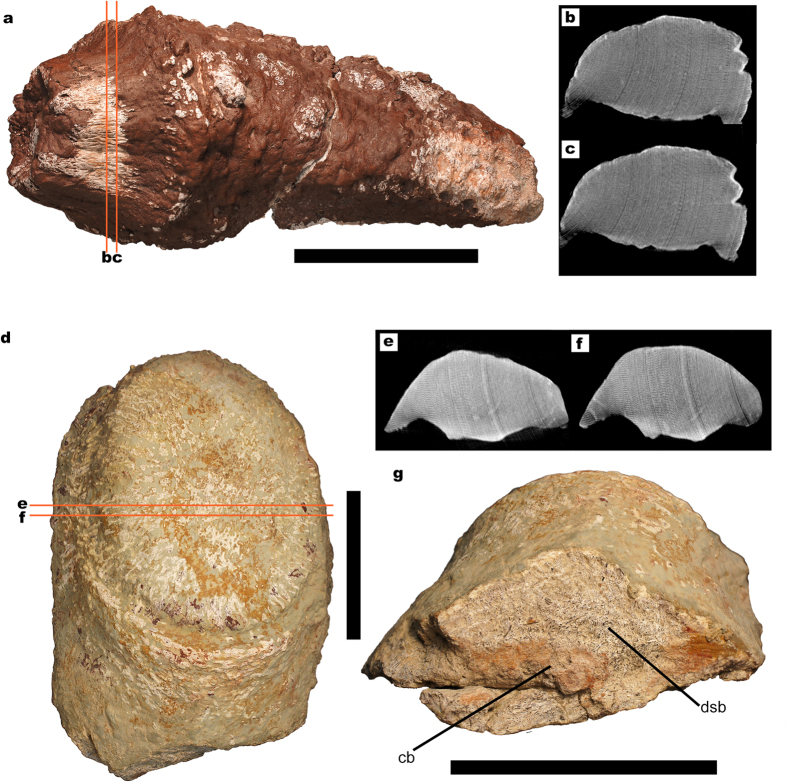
Titanosaur osteoderms without large internal cavities. (a) HUE-02326 in superficial view. (b,c) CT-scan slices at different positions, where no traces of the lower density bone can be observed. (d) HUE-02029 in superficial view. (e–g) CT-scan slices at different positions, where no traces of the lower density bone can be observed. (f) Root-end view of HUE-02029 showing a natural breakage, where most of the section is formed by very dense spongy bone (dsb). In some areas, the spongy bone has collapsed (cb) where longitudinal canals might have been present as in UPUAM-13952. All scales = 100 mm. cb = collapsed bone. dsp = dense spongy bone.
